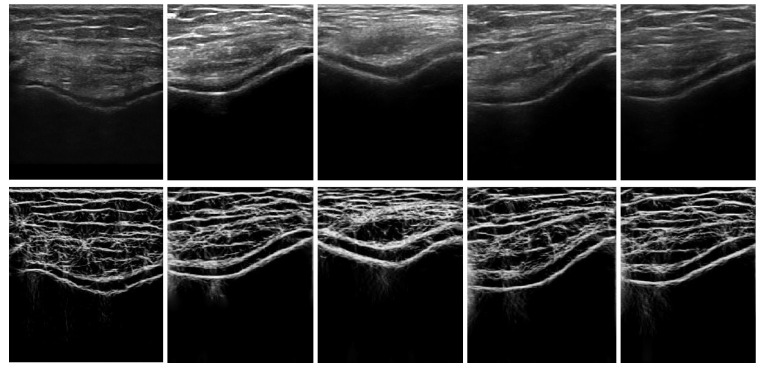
In vivo ultrasound (US) image enhancement: Top row: In vivo B-mode knee-cartilage US image (US(x,y)). Bottom row: Enhanced knee-cartilage US image (USE(x,y)).
